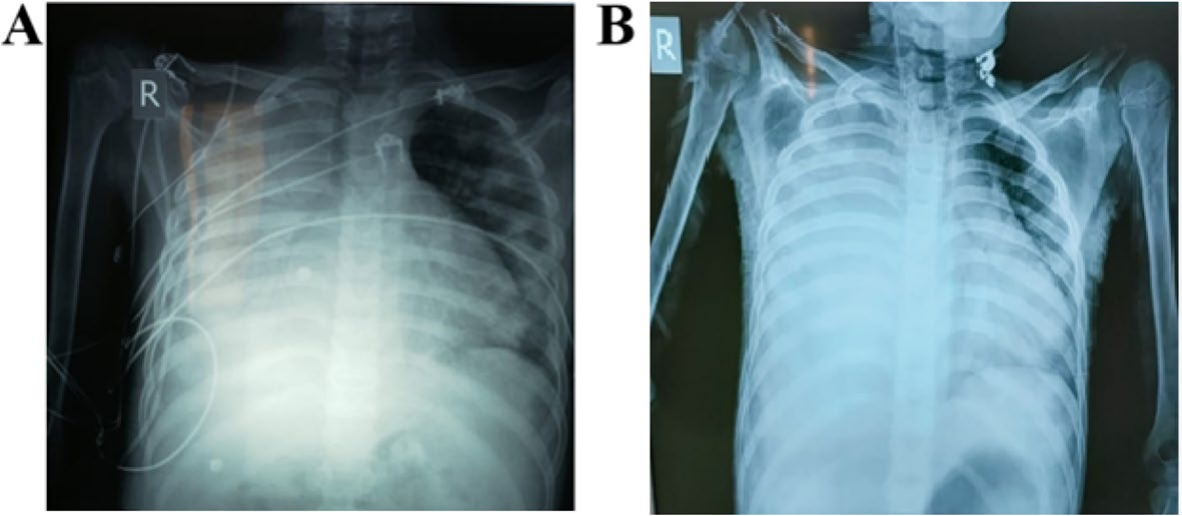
Chest X-ray of the patient showing massive right side pleural effusion. A Pleural effusion after the third day of pleurocentesis on the first presentation. B Reappearance of pleural effusion 4 weeks after first presentation
